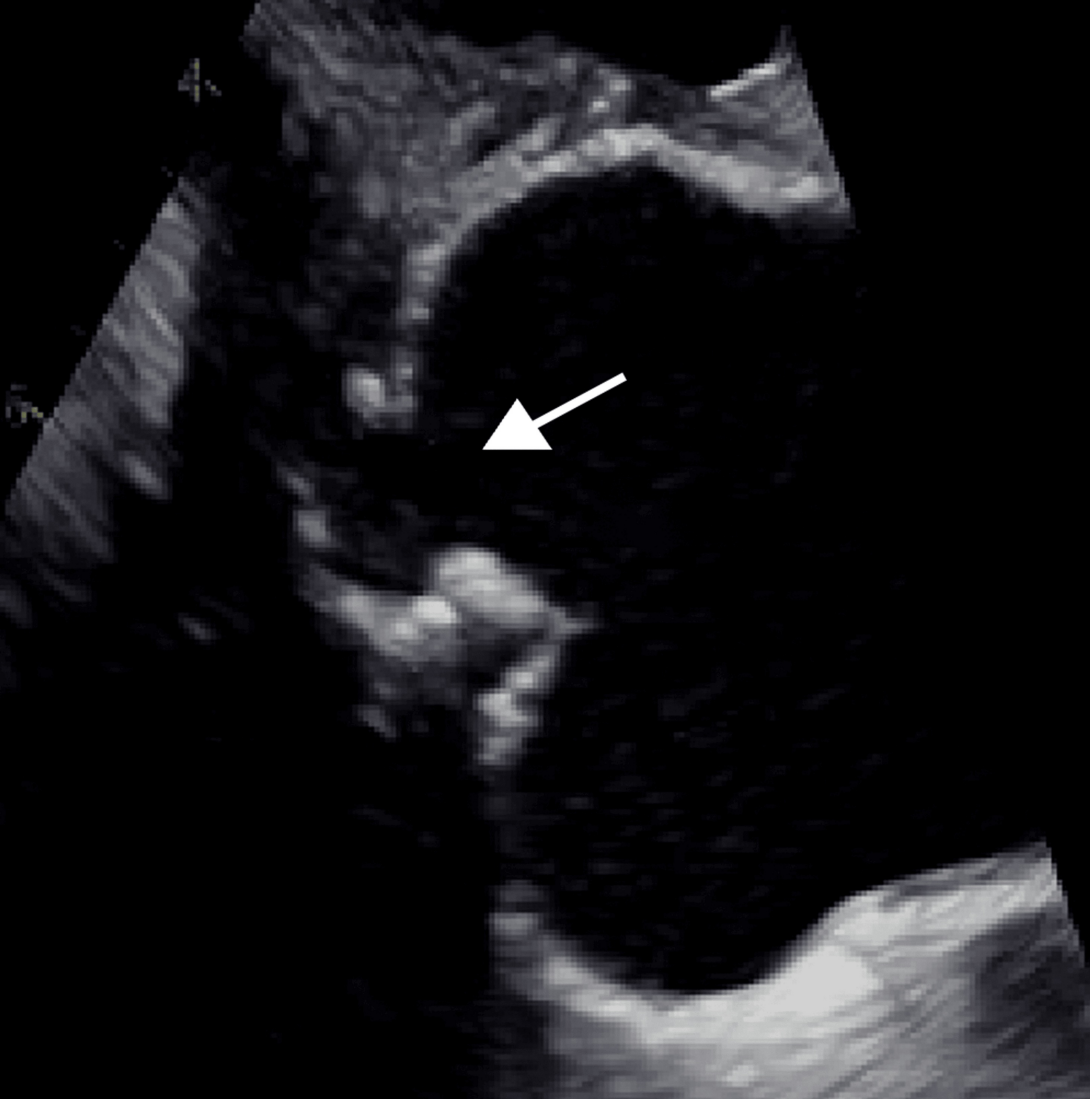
Cardiac MRI showing suspected aortic root aneurysmal outpouching/abscess The arrow shows the outpouching (abscess) on the non-coronary aortic valve cusp
